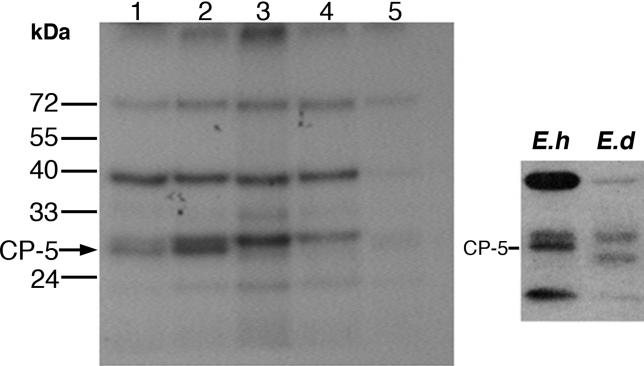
In Vivo Labeling of Cysteine Proteinases The different trophozoite cultures were grown for 18 h with the radiolabeled cysteine proteinase inhibitor Fmoc-[I^125^]Tyr-Ala-diazomethylketone (10 μg/ml, 10 μCi/ml), harvested, washed, and lysed [49]. Samples of each cell lysate (25 μg) were separated on a 12% acrylamide reducing gel and exposed to X-ray film to reveal the different cysteine proteinase bands. Samples in the lanes on the left radiograph are: 1, untransfected HM-1:IMSS; 2, untransfected G3; 3, G3 transfected with pAP-CP5 and grown with 50 μg/ml of G418; 4, RB8 trophozoites after removal of the plasmid (see Results); 5, HM-1:IMSS grown with a lower specific activity inhibitor (0.05 μCi/μg). Samples on the right radiograph demonstrate the CP-5 band location in HM-1:IMSS and its absence from the E. dispar culture [50].
